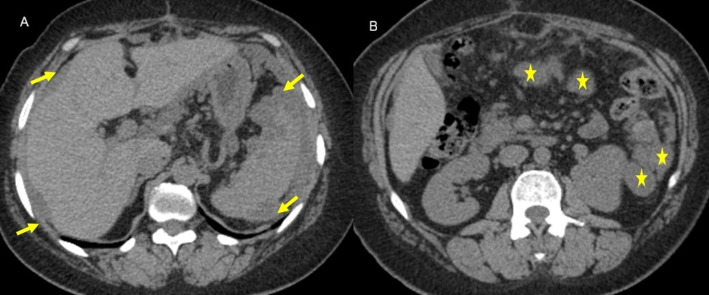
CT of a patient of LAMN with pseudomyxoma peritonei. A 47-year-old woman with pseudomyxoma peritonei and peritoneal deposits. A and B Axial CT images show a relatively high-density fluid at both hypochondrial regions, scalloping both hepatic and plenic surfaces (arrows), and multiple peritoneal deposits, the largest at the left lumbar region measures 3 cm (asterisk)
